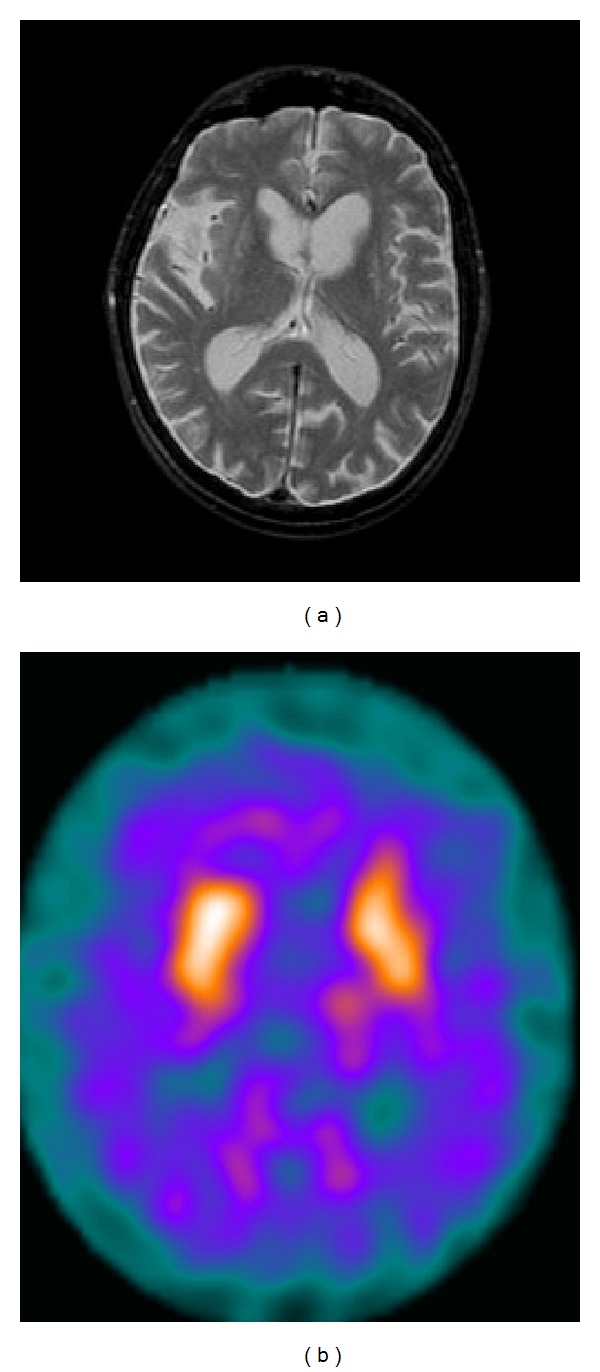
(a) Brain MRI showing severe atrophy of both caudate nuclei and moderate cortical atrophy. (b) SPECT imaging of the striatal dopamine transporter (DAT) with [^123^I]-*β*-CIT revealing a symmetric impairment of uptake in the caudate (mean 0.78, normal range 1.7–3.3) and putamen (mean 0.61, normal range 1.4–3.1).
